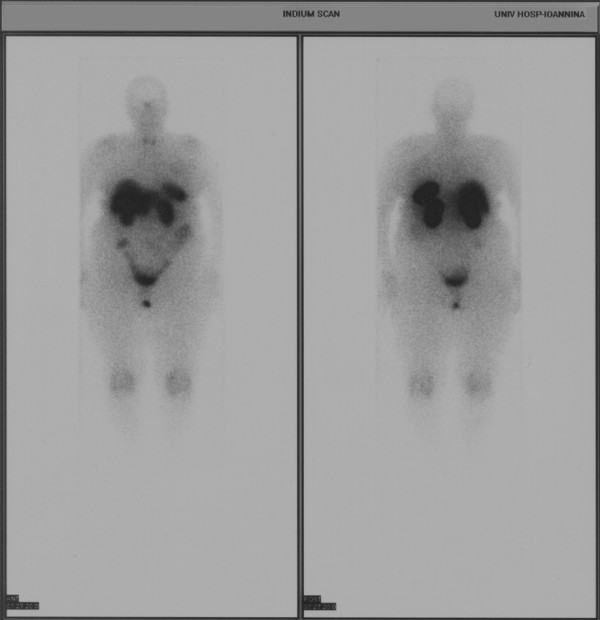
In^111 ^- DTPA - ocreotide scintigraphy. No evidence of increased expression of somatostatin receptors throughout the patient's body was found.
